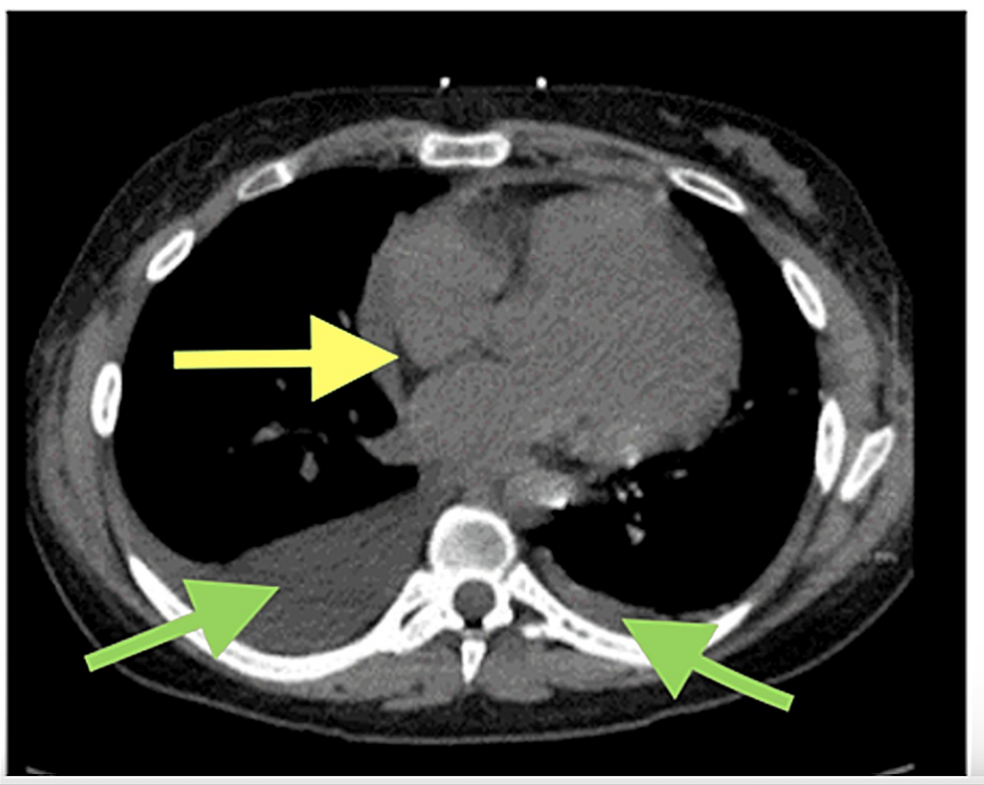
CT without contrast after transverse pericardiocentesis Yellow arrows indicate the pericardial space; Green arrows indicated pleural effusions
